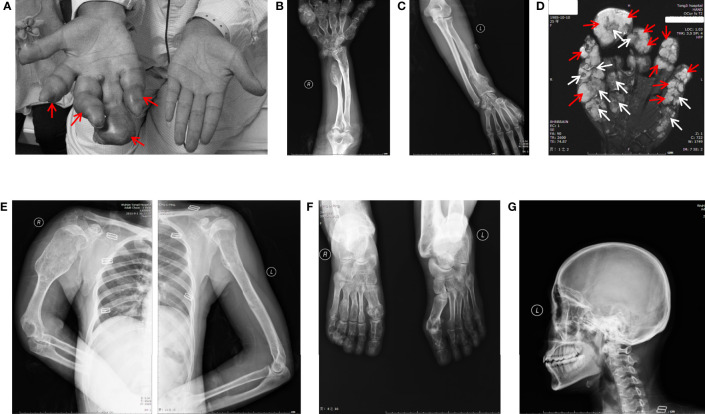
General view and radiographic imaging of the lesion. (A) The right hand of the patient with Maffucci’s syndrome showed deformities due to multiple enchondromas and a superficial hemangioma. The red arrows show hemangioma as a soft spherical bulge in light blue. (B) X-ray images of the right ulnar and radial bones and multiple metacarpophalangeal bones of the right hand show multiple reduced bone densities and partial expansive bone destruction (C). The X-ray shows a relatively normal bone mass in the left arm. (D) MR results of the patient’s right hand showed the phalanges are circular or oval, multilocular, well-defined, iso-intensity and hyperintensity on T2WI (white arrows) and the soft tissue around the phalanx presents dilatative, well-bounded hyperintensity on T2WI (red arrows). (E) X-ray images of the patient’s upper body and enlarged right arm are shown in the film. (F) X-ray images of the patient’s feet have the same radiographic appearance as those of the upper limbs. (G) X-ray image of cranial and maxillofacial bones showed no apparent abnormalities.
